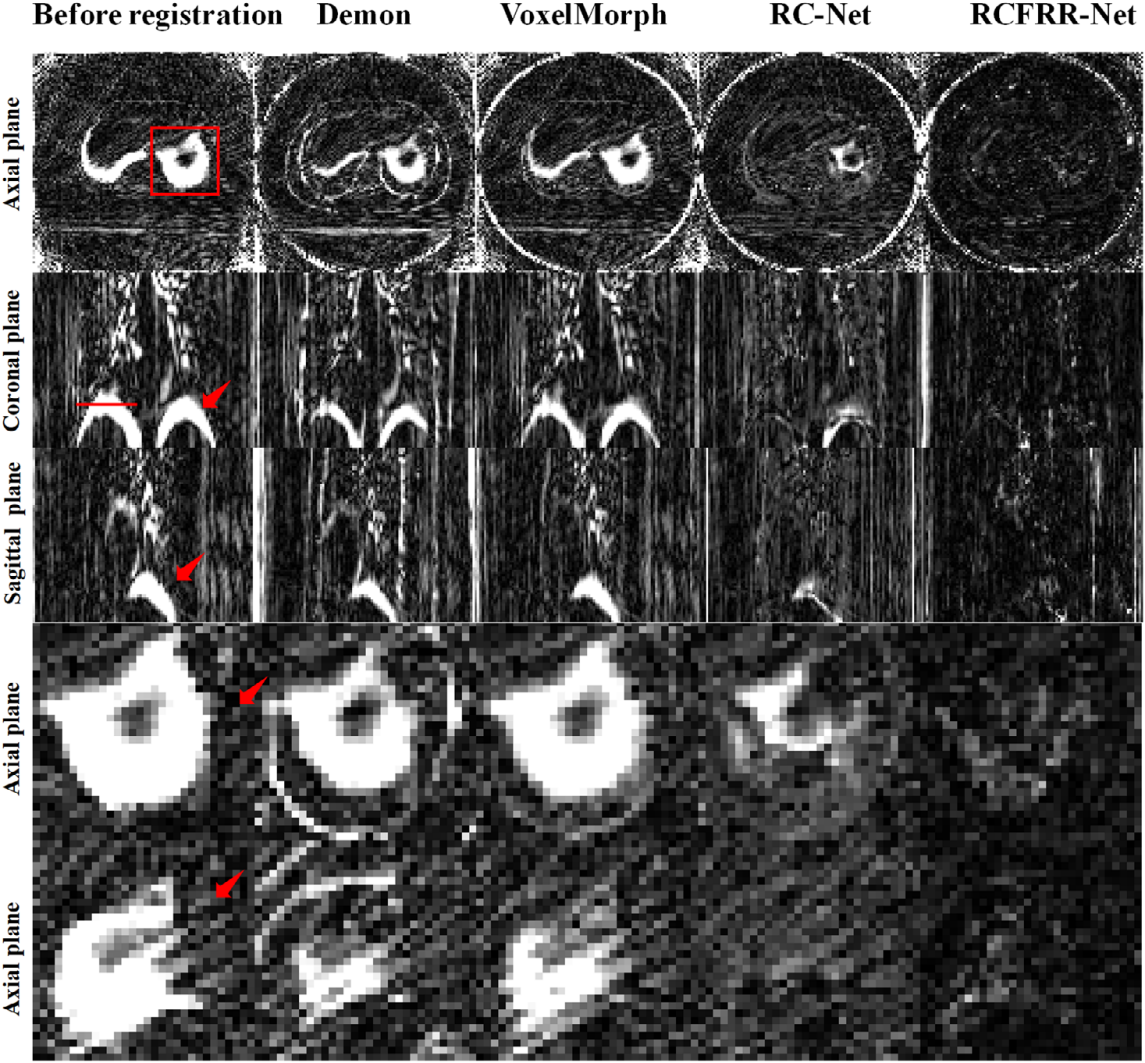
Comparison of the residual images between the fixed images and warped images of different registration methods on 4D‐CBCT datasets. Display window: [0, 400] HU.
